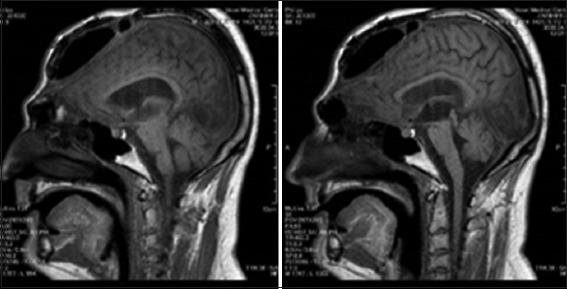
Magnetic resonance images obtained after bone flap fixation
